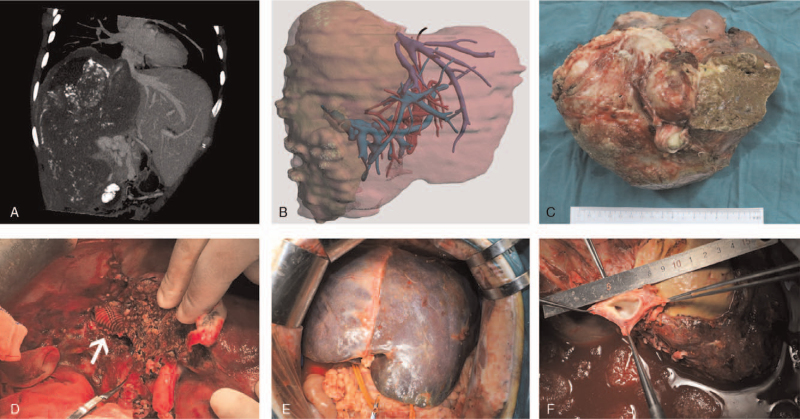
Preoperative assessment and surgical procedures for ex vivo liver resection and autotransplantation. (A) Preoperative CT scan shows a massive hepatic alveolar echinococcosis lesion occupying the entire right lobe and left medial lobe. (B) A 3D reconstruction of the patient's liver ^[29]^. (C) The lesion is approximately 20 cm × 15 cm; (D) An artificial graft was used to lengthen the outflow tract to prevent potential postoperative kinking. (E) Congestion of the residual liver. (F) The left hepatic vein has only 1 small hole left in it.
